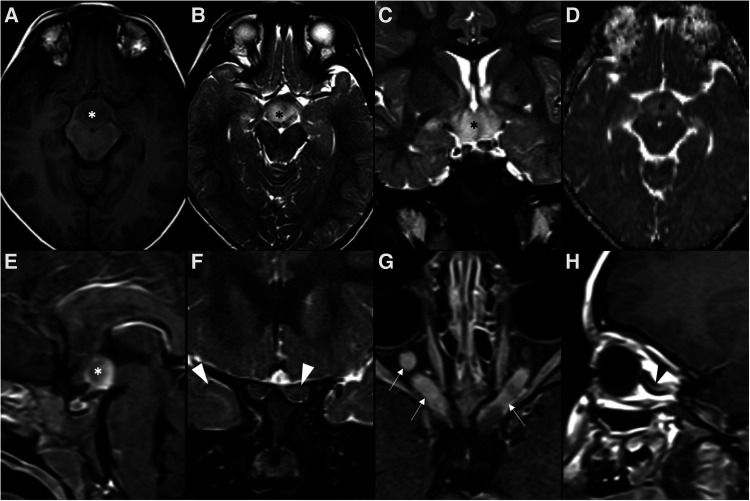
Neurofibromatosis type 1-related hypothalamic and optic pathway astrocytoma in a 3-year-old boy. Brain MRI axial T1WI (A), and axial (B) and coronal (C) T2WI show a T1 hypointense and T2 hyperintense suprasellar infiltrative neoplasm (asterisks), with facilitated diffusion on ADC map (D) and solid enhancement on sagittal T1WI after paramagnetic contrast media injection (E). Coronal T2W images with fat suppression (E) depict anterior extension to both optic nerves, with T2 signal change and increased thickness (white arrowheads). Enhancement (white arrows) and downward kinking (black arrowhead) of the orbital segment of the optic nerves can be seen on axial (G) and left paramedian sagittal (H) T1WI, respectively. Several focal areas of signal intensity (FASI), including on the left globus pallidus (black arrow on C), were detected.
